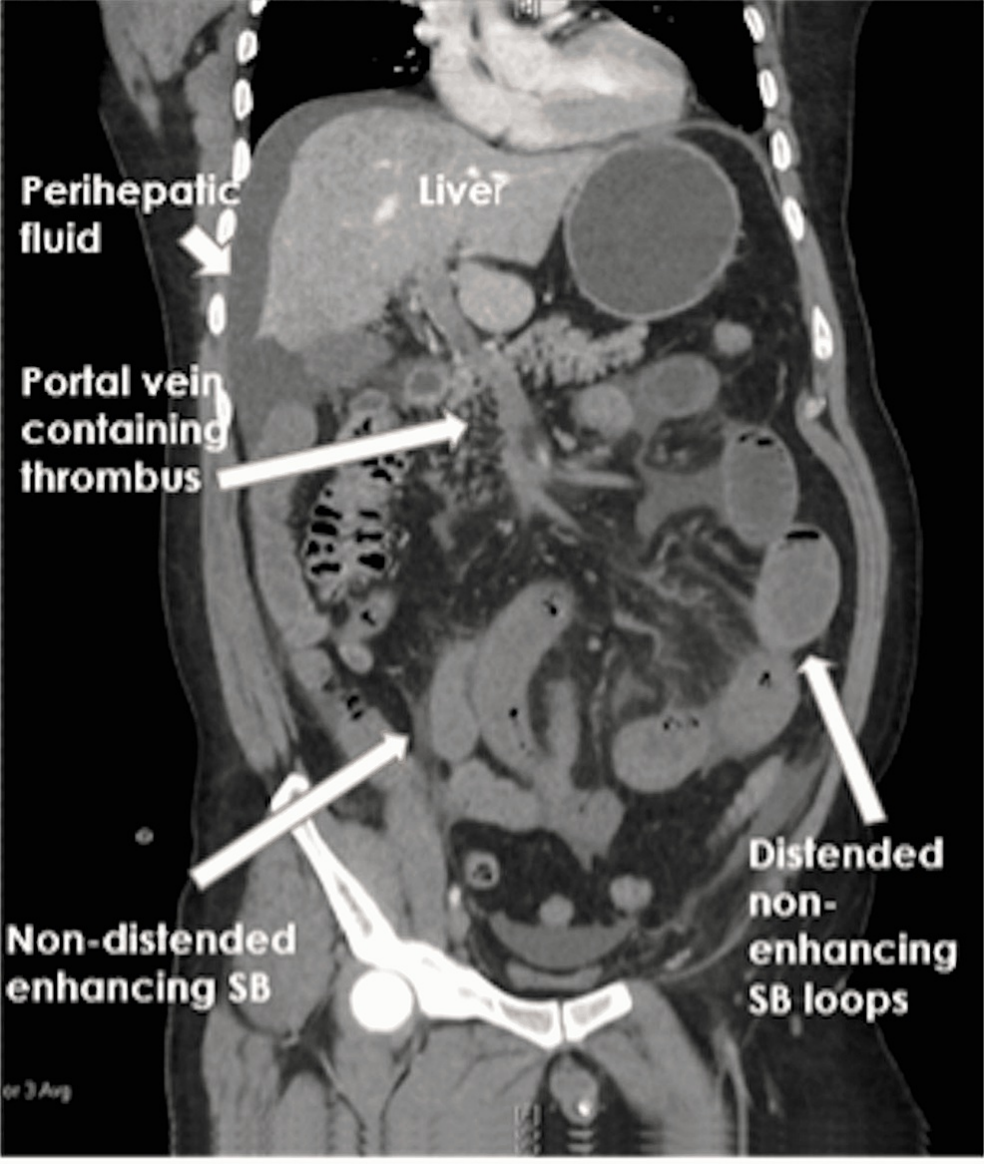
CT Abdomen showing venous thrombus and ischaemic bowels.
